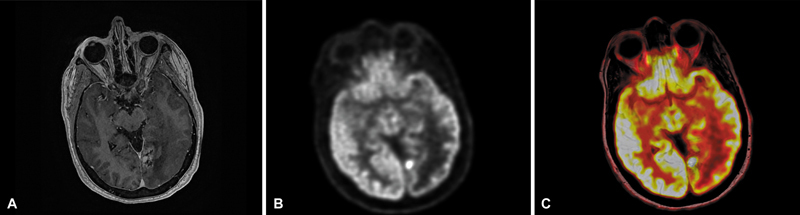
Axial T1-weighted post-contrast image of a left occipital lesion (
